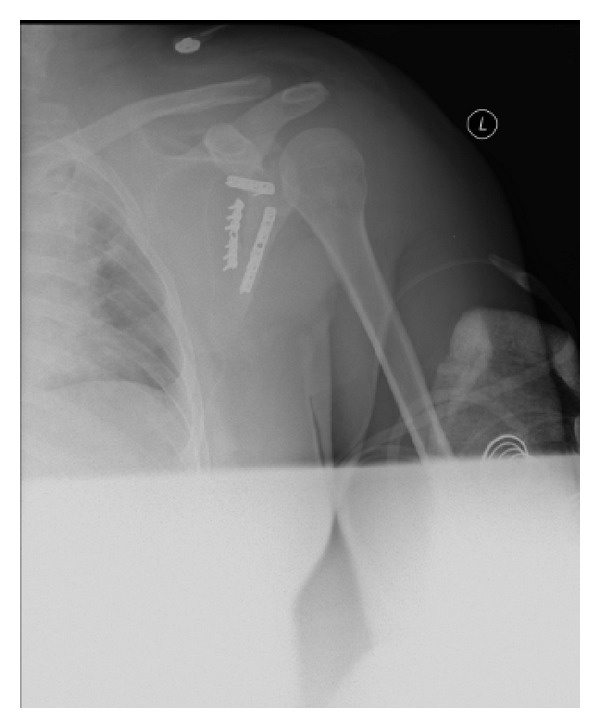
Postoperative anteroposterior radiograph of the left shoulder demonstrating an anatomic reduction of the scapular fracture with good positioning of the implants.
